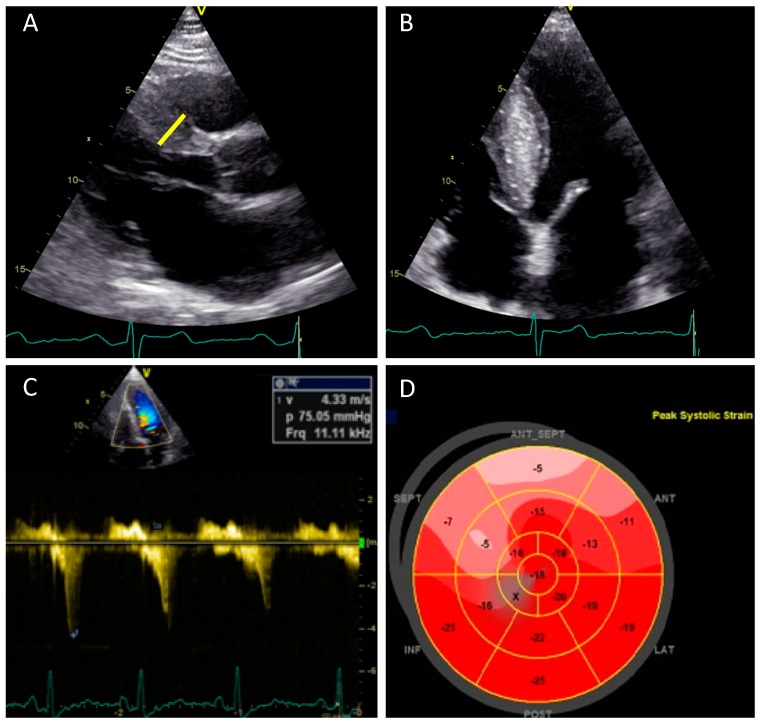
The role of transthoracic, two-dimensional echocardiography in the assessment of hypertrophic cardiomyopathy (HCM). (A) A parasternal long axis view showing basal septal hypertrophy (maximum LV wall thickness 21 mm—yellow line). (B) Apical four-chamber view shows hypertrophy of the interventricular septum. (C) Continuous wave Doppler from modified apical five-chamber view showing a rest dynamic gradient of 75 mmHg in the LVOT (D) A bull’s eye plot of two-dimensional speckle tracking showing reduced peak longitudinal strain values in the septum—the areas most affected by hypertrophy with preservation of the basal-to-apical gradient. HCM—hypertrophic cardiomyopathy, LV—left ventricle, and LVOT—left ventricular outflow obstruction.
